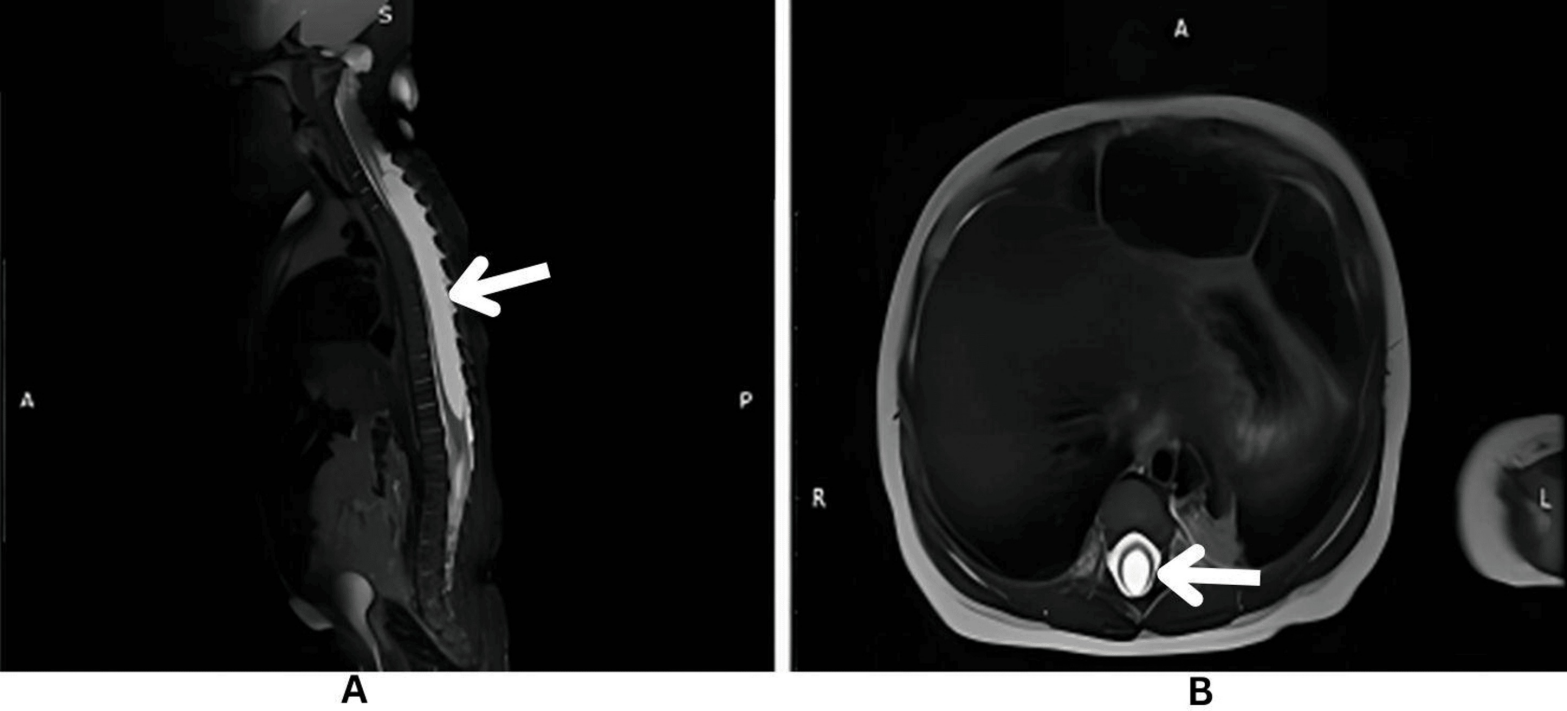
A. MRI sagittal T2 fat-suppressed sequence of the spine showing a fluid-filled cavity (white arrow) in the cervico-thoracic spinal cord. The cavity is continuous and centrally located, and the features are suggestive of a syrinx. B. MRI axial T2-weighted sequence of the spine showing a centrally located fluid-filled cavity (white arrow). The features are suggestive of a syrinx.
